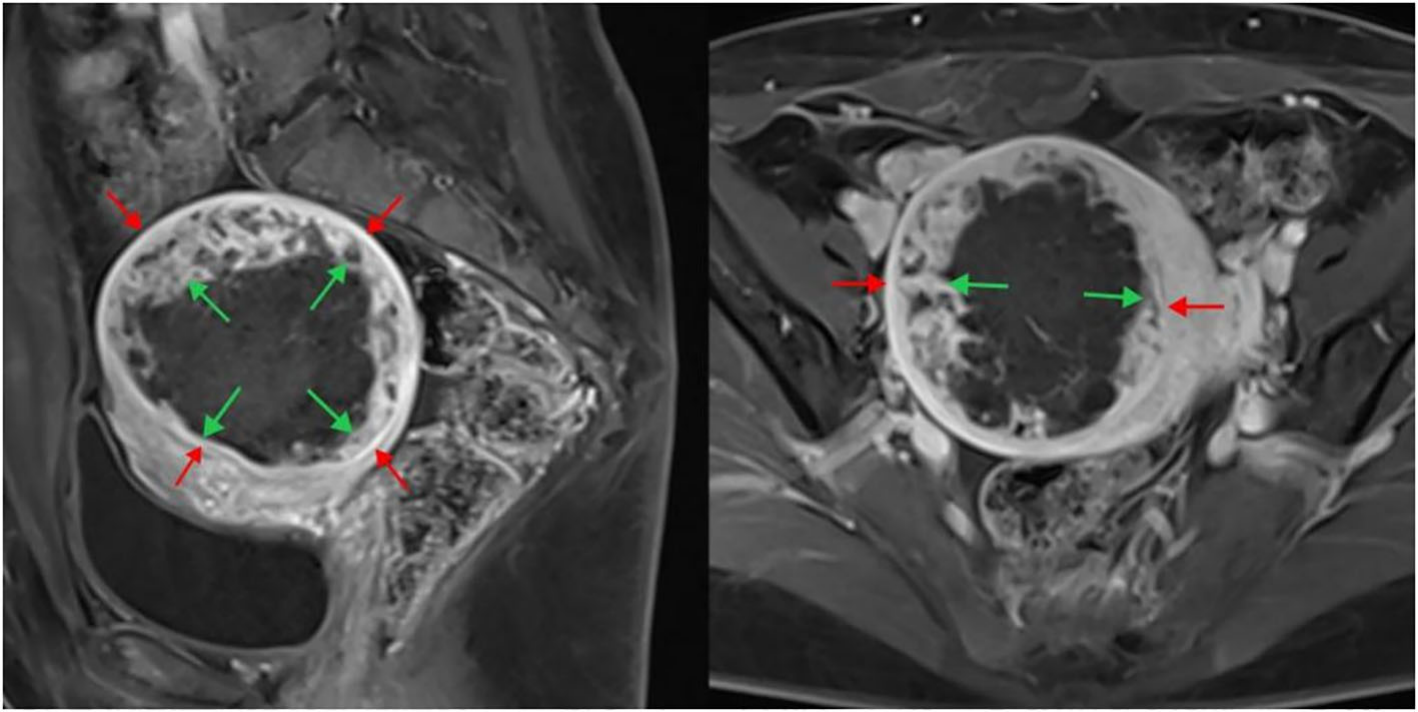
Enhanced MRI image after HIFU treatment of uterine fibroids. The sagittal plane is shown on the left, and the cross-section is shown on the right. The inner arrow indicates the size of the non-perfused area, and the outer arrow indicates the fibroid size. HIFU, high-intensity focused ultrasound; MRI, magnetic resonance imaging.
